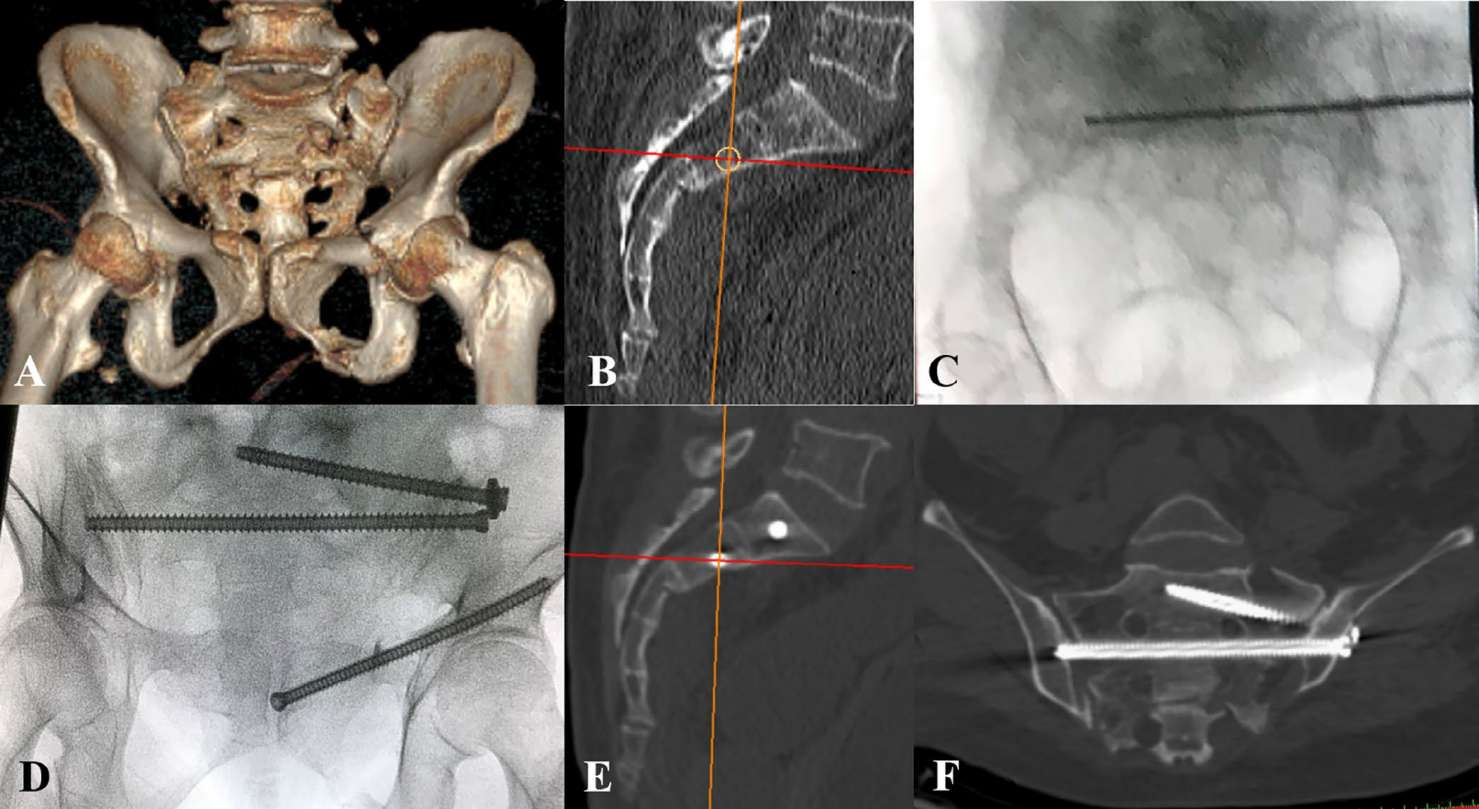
A 69‐year‐old woman suffered a minimally displaced pelvic fracture (OTA‐61C1.3) (A). The optimal entry point and the safe osseous corridor (orange circle) for transiliac‐transsacral screw of S2 were formulated in the strict mid‐sagittal plane (B). A reversed Kirschner wire was placed along the percutaneous kyphoplasty canula (C). Postoperative pelvic anteroposterior radiograph, strict mid‐sagittal CT image, and sacral coronal CT image showed the Grade‐2 placement of the screw (D–F).
